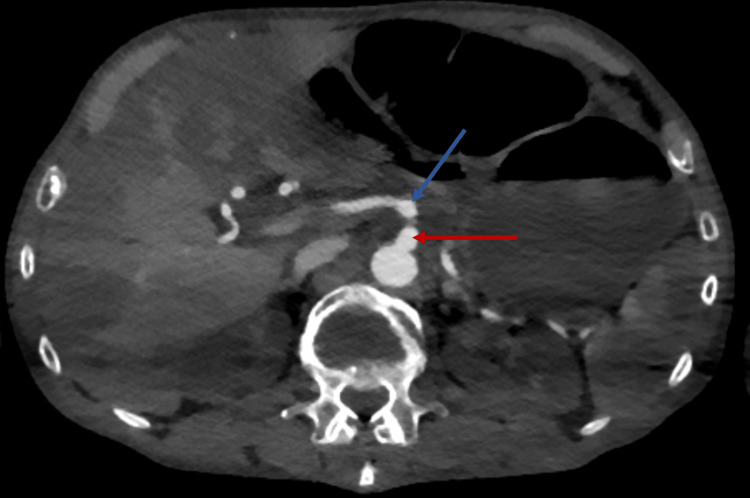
Abdominal CT scan with angiography. Blue arrow shows the superior mesenteric artery. Red arrow indicates the celiac trunk. Both arteries are filled with contrast, suggesting open vessels.
